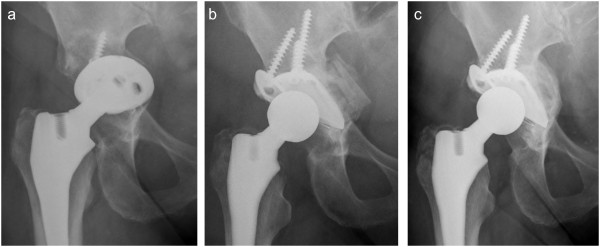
a. Preoperative anteroposterior radiograph of a 72 year old woman, 13 years after total hip replacement, showing aseptic loosening of the acetabular component with migration into the small pelvis (type III B). b. 7 days after acetabular revision using a Mueller ring and fresh frozen non-irradiated allograft bone. Note the distinguishable allograft bone chips medial of the ring. A polyethylene cup was cemented into the ring. c. 28-month follow up radiograph showing the implants to be unchanged with complete remodeling of the allograft bone and homogeneous trabeculation.
